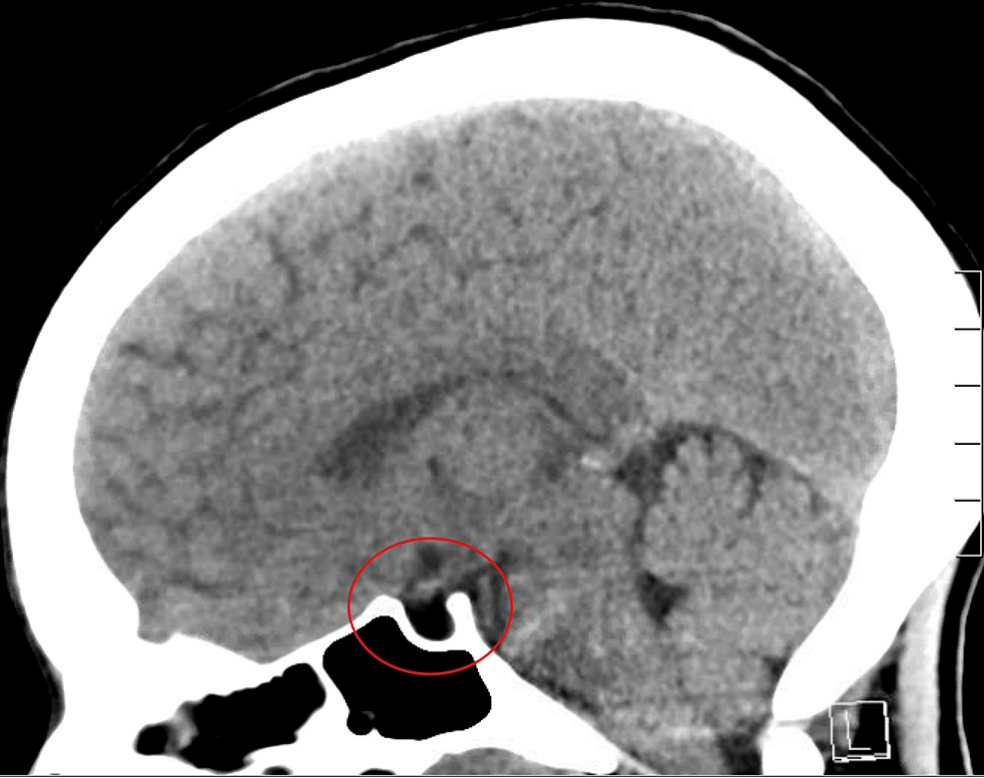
Computed Tomography (CT) without contrast of the head for this patient showing absence of appropriate anatomical structures within the sella turcica
